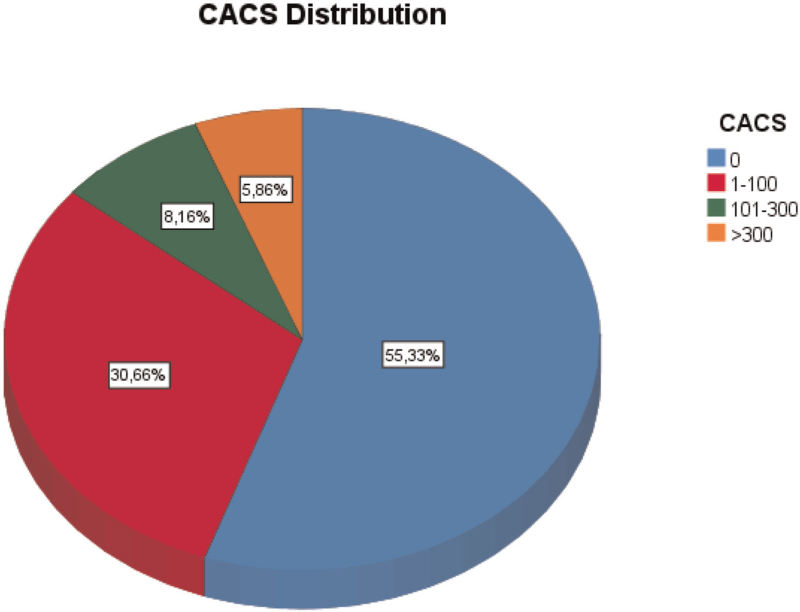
Distribution of coronary artery calcification score in the study population. Distribution of coronary artery calcification score in the study population. The score corresponds to Agatson score measured by computed tomography. CACS, coronary artery calcification score.
